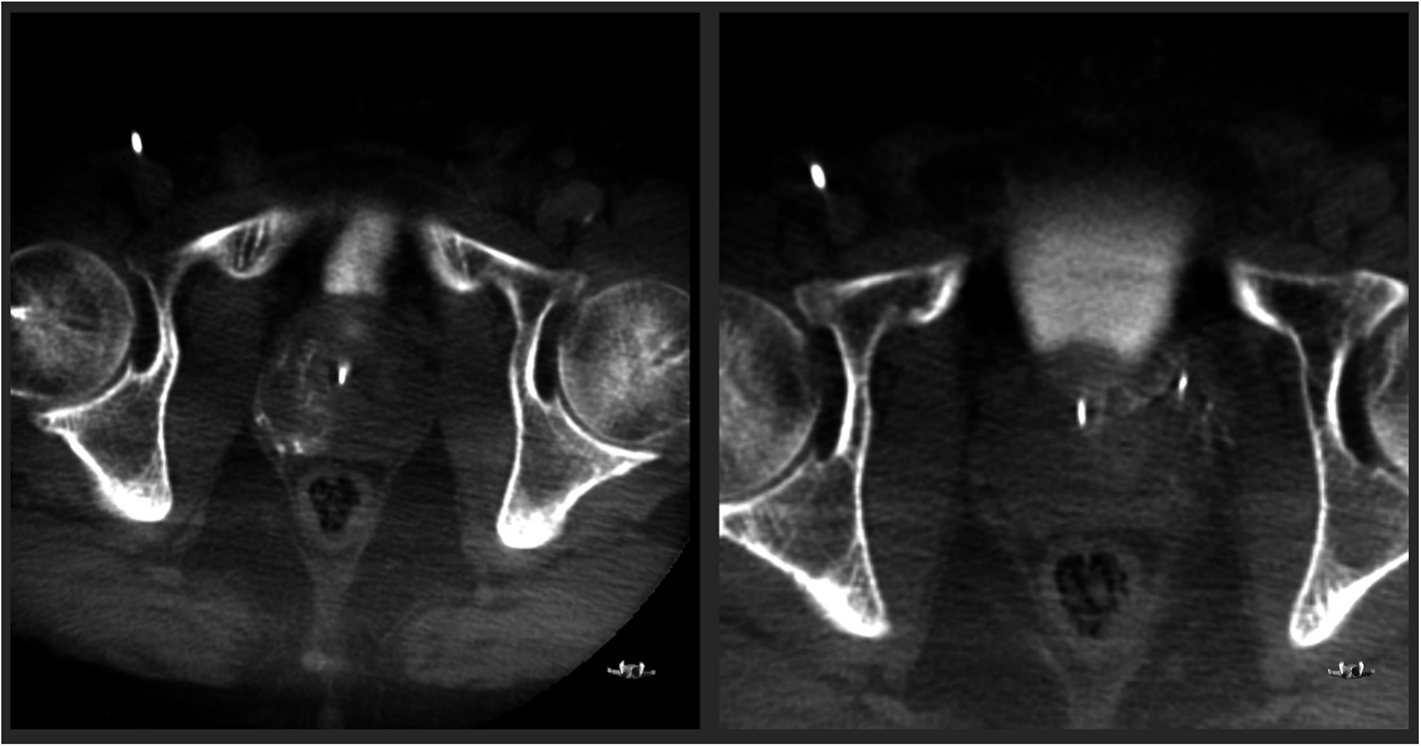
Cone beam CT (CBCT) images from Patient 2 used for confirmation of microcatheter position show contrast enhancement in right (left panel) and left (right panel) prostate sides. No enhancement is seen in the neighboring structures
